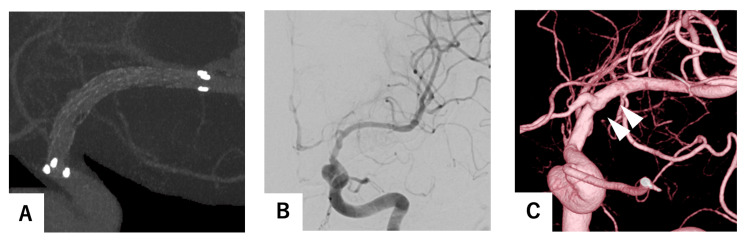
One-year follow-up angiography after the endovascular surgical procedure. (A, B) Cone-beam computed tomography demonstrated adequate stent expansion. (C) Cerebral angiography revealed no stenosis and maintained vascular patency. Additionally, three-dimensional rotational angiography indicated that the stenotic lesion in the internal carotid artery remained dilated.
